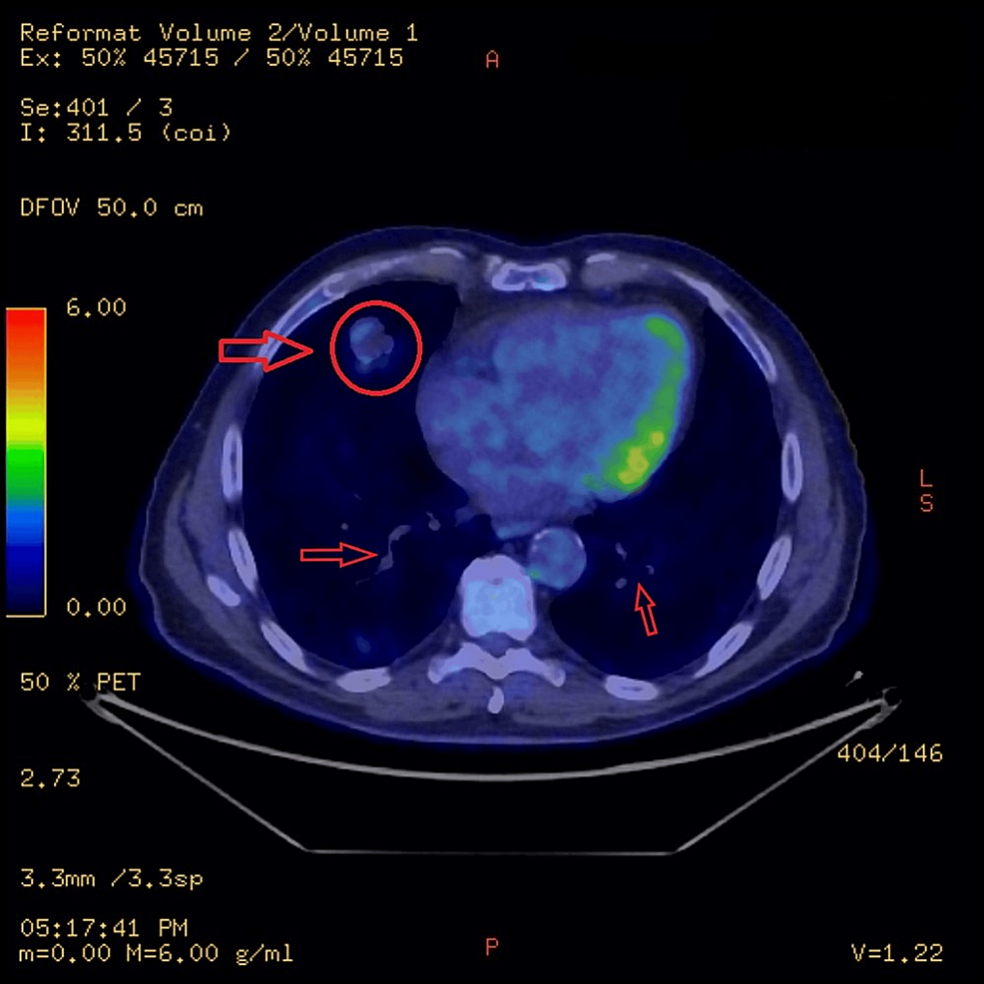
FDG uptake in the lung nodules
